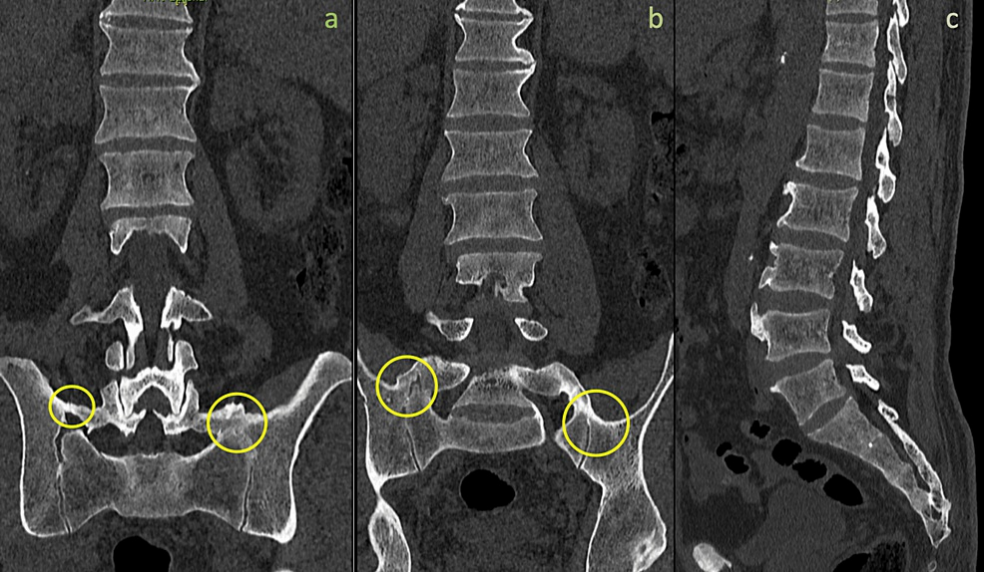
Coronal view (a and b) of lumbosacral spine CT revealing bilateral transverse mega-apophysis of L5 (yellow circles) articulating with the sacrum and ilium along with lumbar osteophytes. Sagittal view (c) detecting lumbar osteophytes CT: computed tomography
